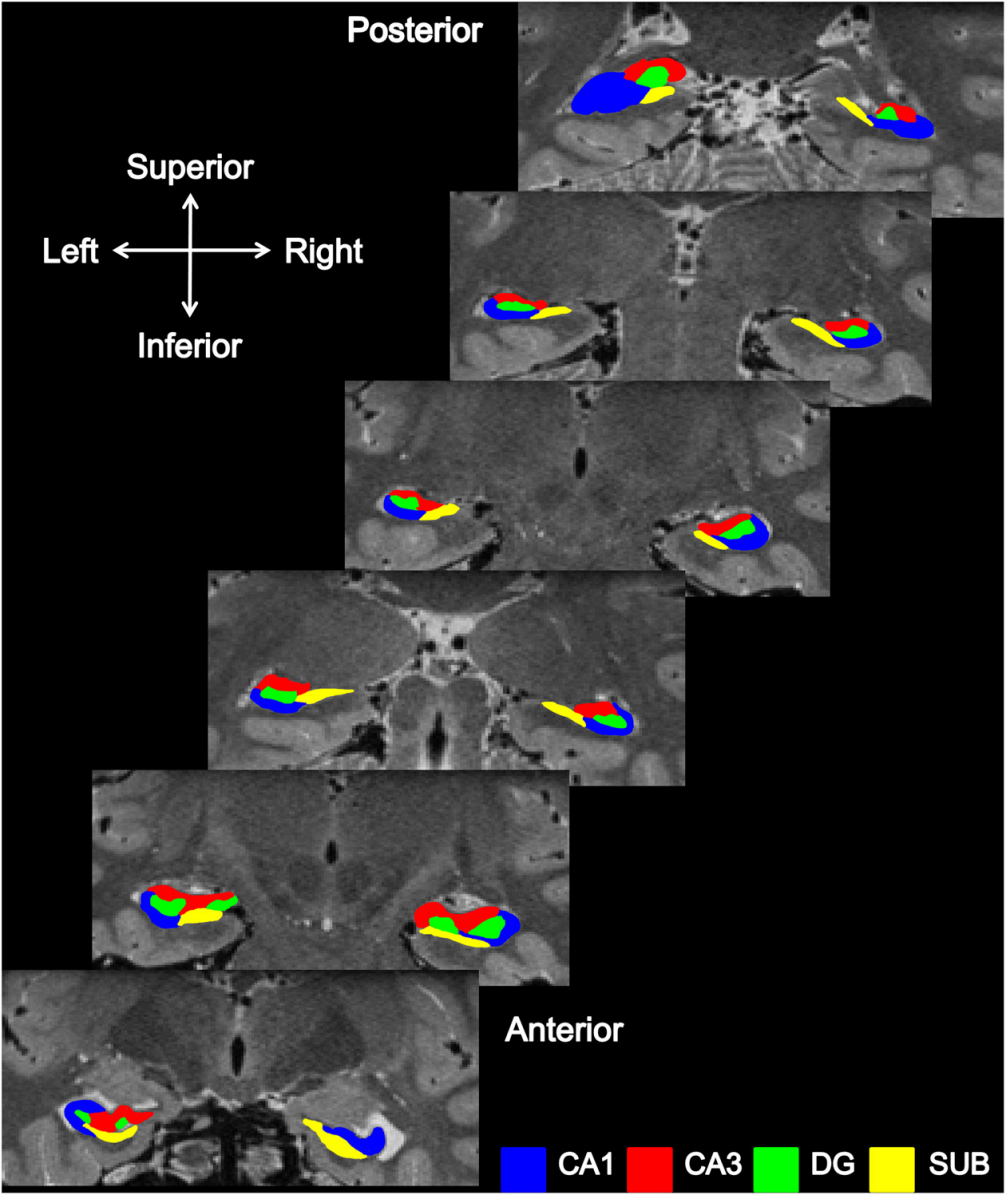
Subfield segmentation in the coronal plane. Coronal sections through an averaged T2-weighted image of both the left and right hippocampus of a participant.
